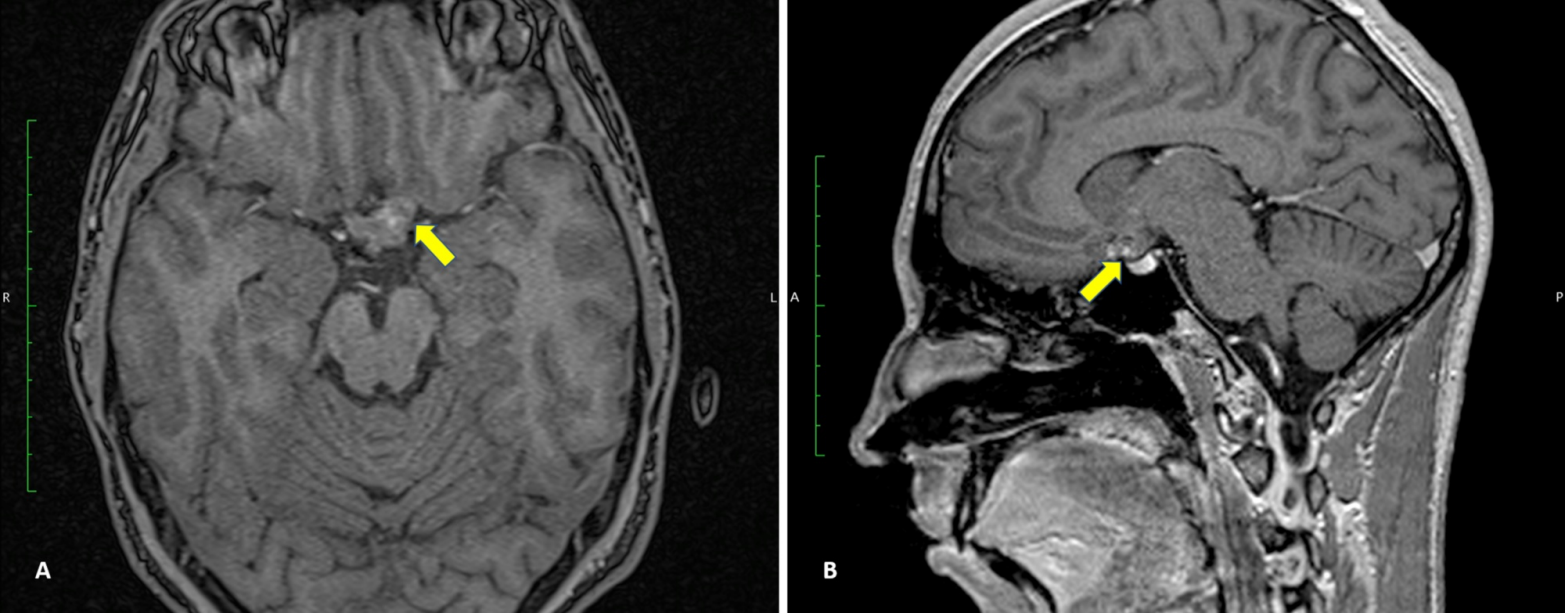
Preoperative (A) axial and (B) sagittal MRI. Hyperintense signal on T1-weighted MRI showing a mass lesion occupying the chiasm and left optic nerve.
